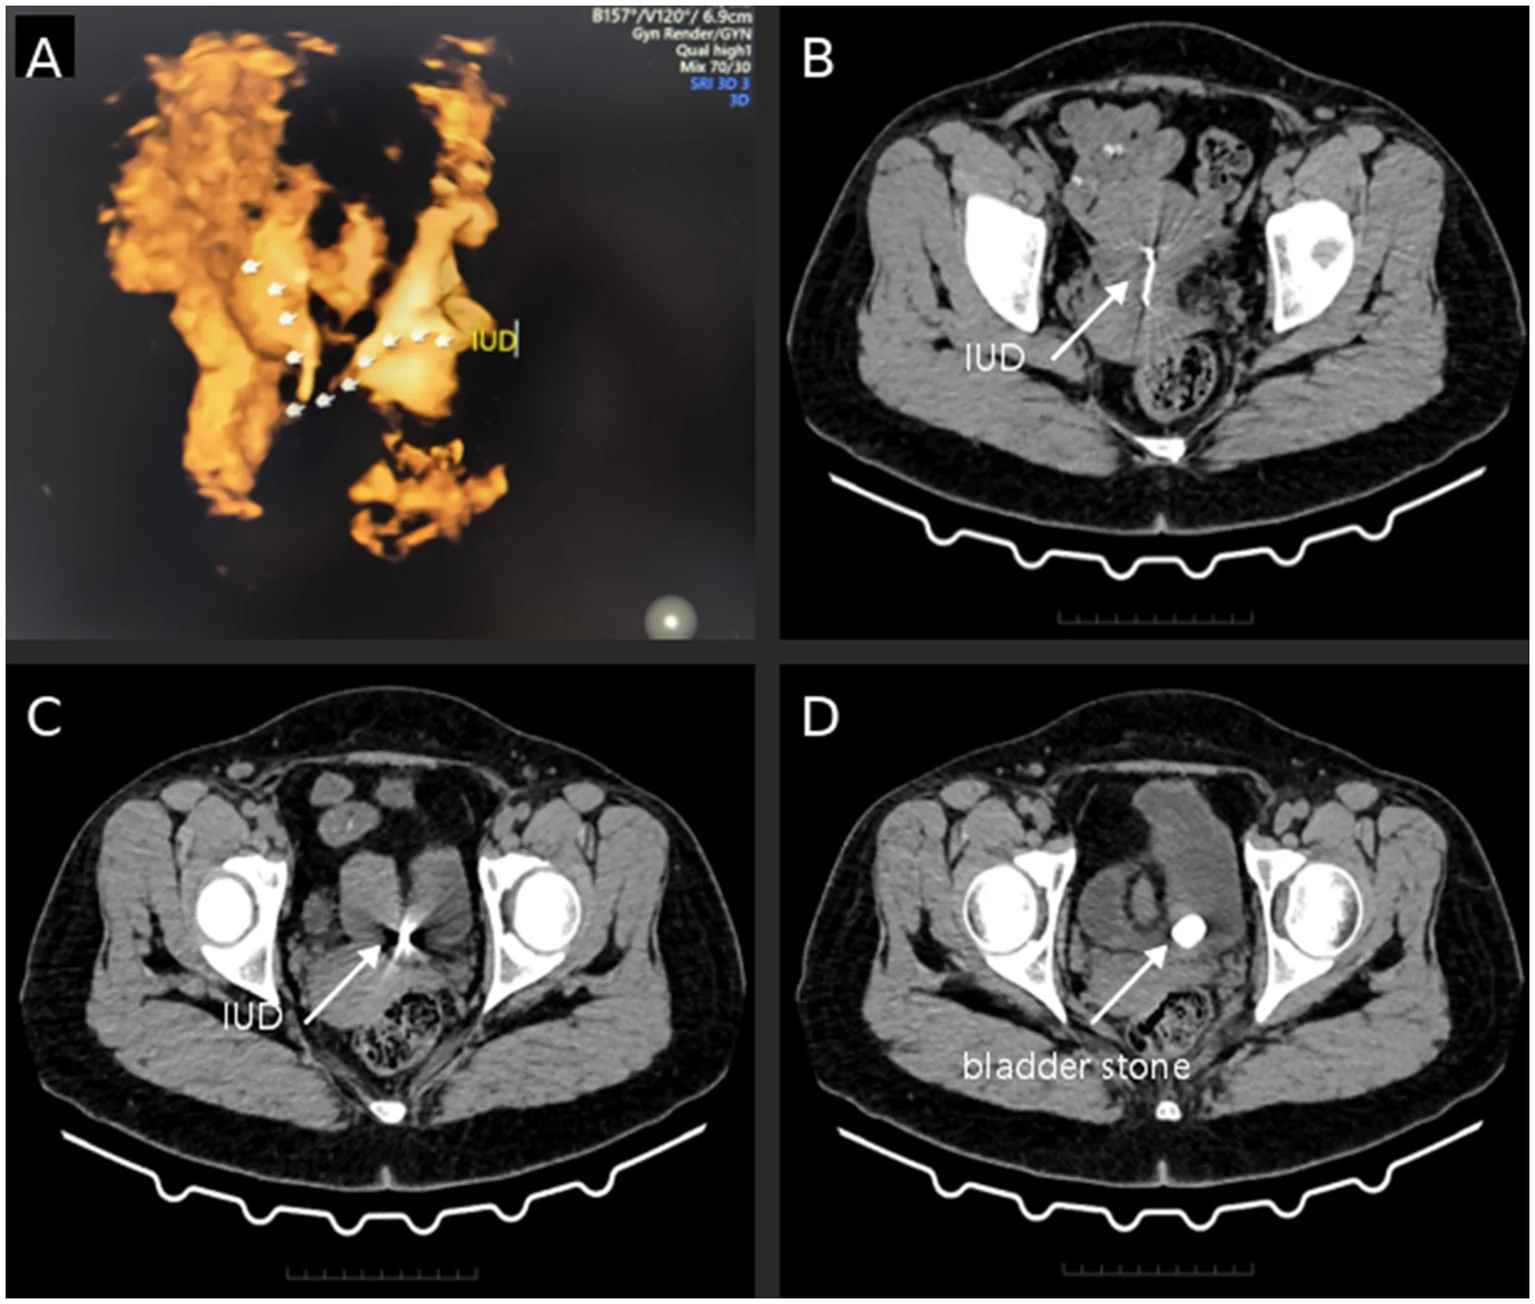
Preoperative ultrasound and CT findings demonstrating IUD migration into the bladder with secondary bladder stone formation. (A) Ultrasonography demonstrating the majority of the IUD within the endometrial cavity, with one arm penetrating the myometrium and projecting toward the bladder. (B) Axial CT image revealing a linear hyperdense IUD (white arrow) within the uterine cavity. (C) Axial CT image demonstrating one IUD arm (white arrow) traversing the uterine wall and entering the bladder lumen. (D) Axial CT image showing a hyperdense bladder calculus (white arrow) encasing the distal IUD arm.
